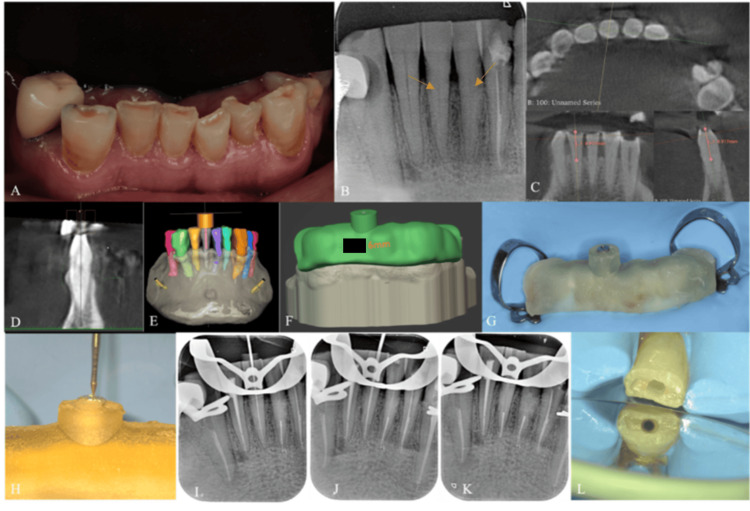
(A) Intraoral photo showing tissue loss due to severe bruxism on the anterior teeth. (B) Initial preoperative X-ray showing calcified root canals on teeth 31 and 41. (C) Measurement of calcification level on 3D slicer. (D) Advanced semi-automatic segmentation of tooth 41 and customized virtual implant presenting the drill’s position. (E) Virtual drill-sleeve’s location. (F) Virtual template of the guide with the patient’s initials and the length of the sleeve to be used engraved on it. (G) Endodontic guide placed after ensuring perfect isolation using a rubber dam. (H) Drilling through the endodontic guide and sleeve, drill of 0.28 mm tip diameter (SSWhite Dental). (I) Working length determination. (J) Cone fit. (K) Post-obturation of the apical 5 mm. (L) Guided access cavity preparation.
